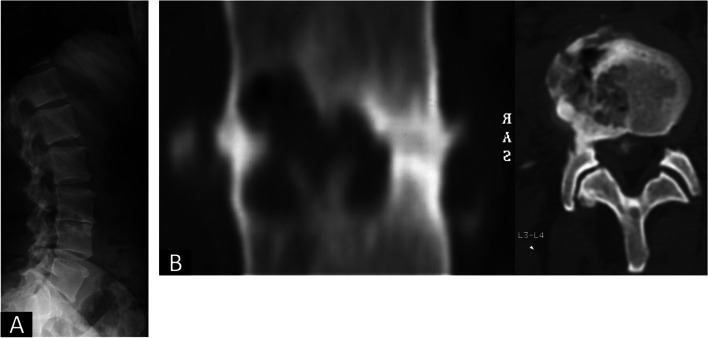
A and B: The imaging obtained in September 2003 showed a rapidly evolving degeneration of the L3/4 disk space (A) with an erosion of the L3 and L4 endplates in the CT scan (B)
